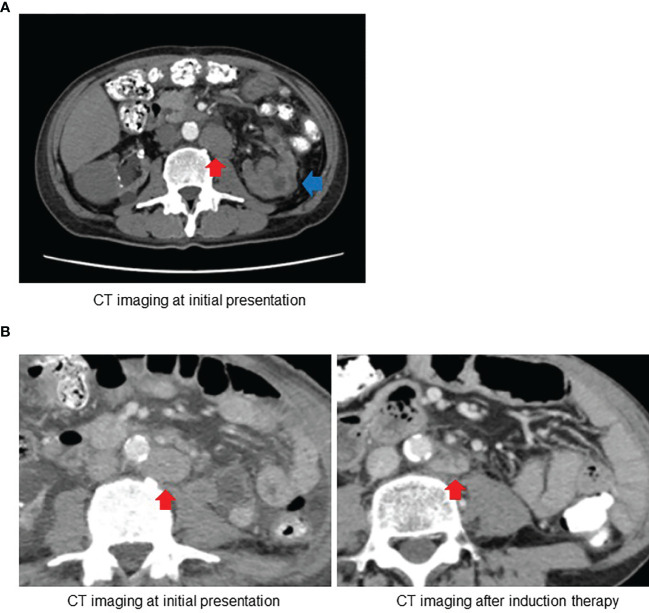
(A) Computed tomography (CT) at initial presentation revealed the left kidney tumor (blue arrow) with para-aortic lymph node involvement (red arrow). (B) Follow-up CT after induction therapy with ipilimumab and nivolumab showed substantial decrease in size of para-aortic lymph node.
